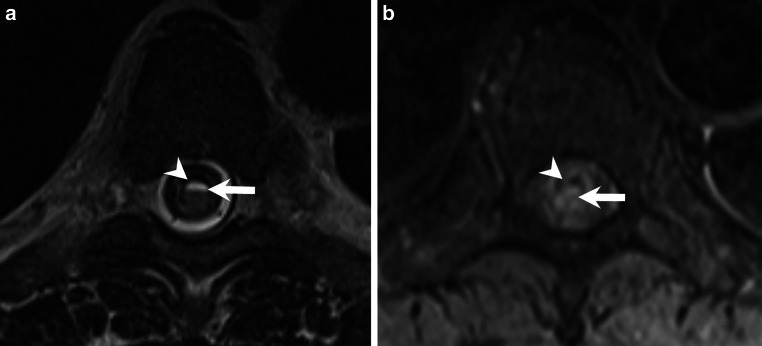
Axial T2-weighted images show the cystic lesions with a fluid-fluid level (a, arrow) and a pronounced hypointense rim (a, arrowhead). Corresponding axial non-enhanced T1-weighted images at the same level reveal a slightly hyperintense signal relative to the spinal cord (b, arrow) and a hyperintense rim (b, arrowhead)
